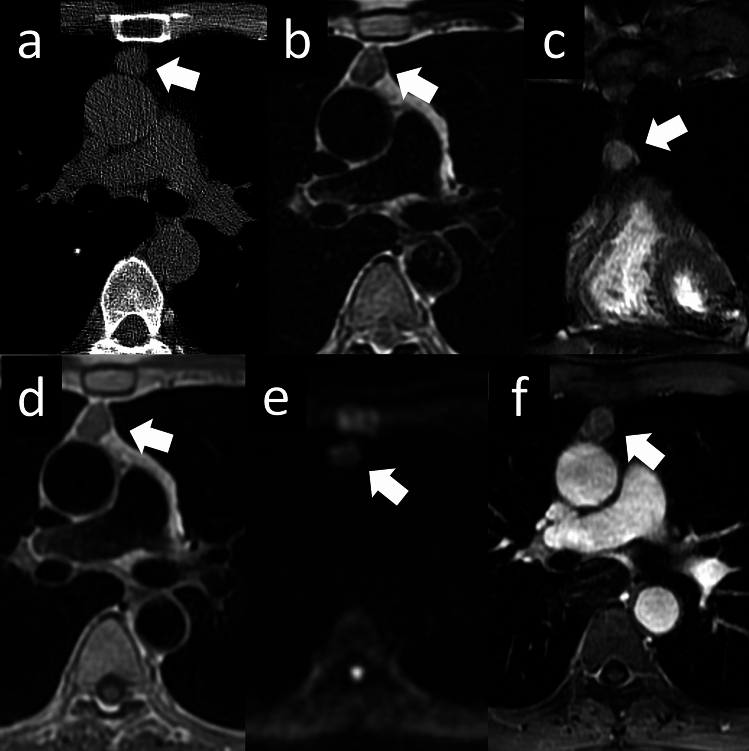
Thymic neuroendocrine neoplasm. A 50-year-old woman with a thymic neuroendocrine neoplasm. a Chest CT scan shows a 2.0-cm nodule (arrow). b–d MRI shows heterogeneous signal intensity on T2WI (b: axial, c: coronal) and T1WI (d). e MRI shows slightly high signal intensity on DWI (arrow). f MRI shows a slight enhancement on contrast-enhanced T1WI (arrow). CT, computed tomography; MRI, Magnetic Resonance Imaging; T2WI, T2-weighted imaging; T1WI, T1-weighted imaging; DWI, diffusion-weighted imaging
